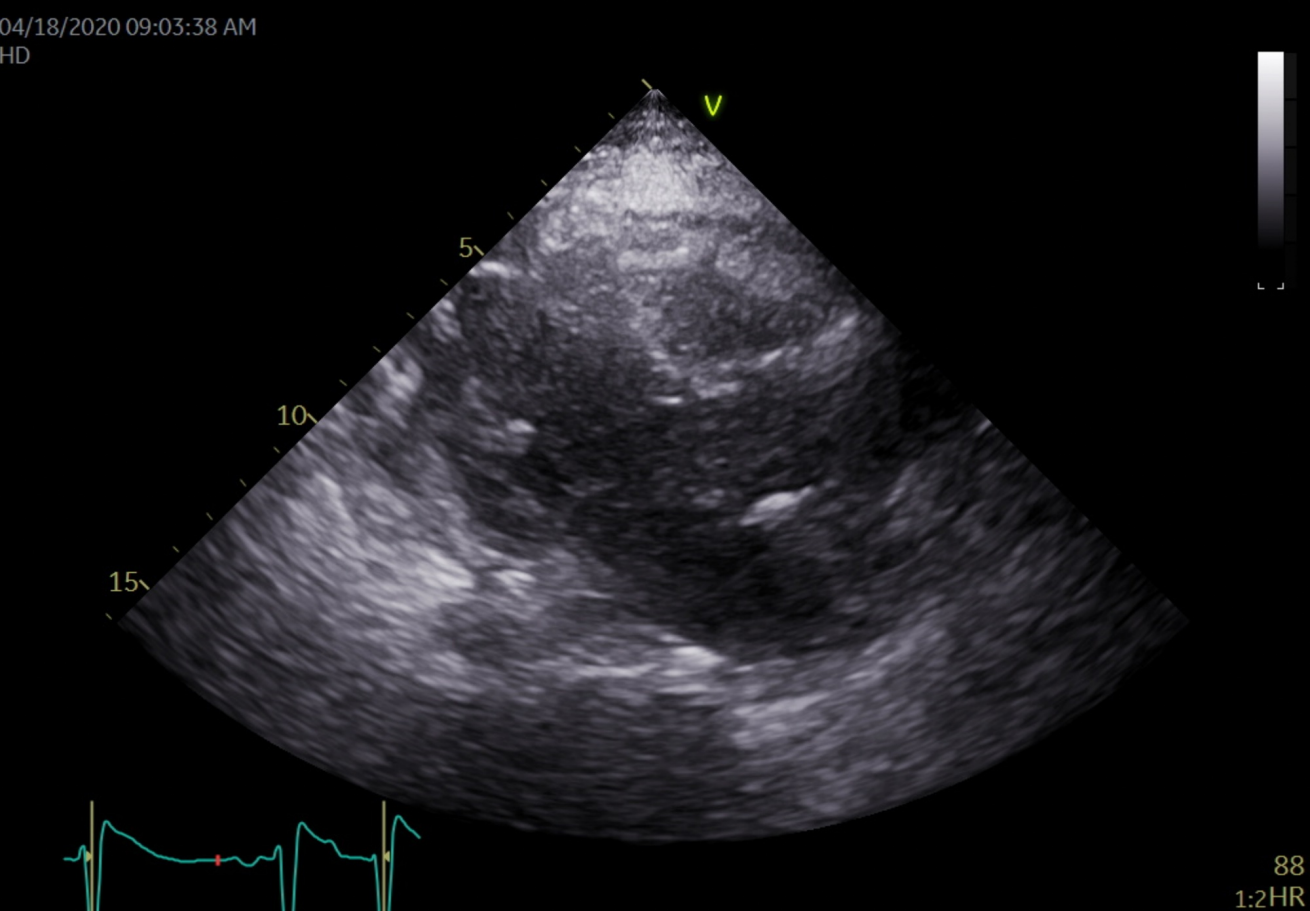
A parasternal long axis view showing the presence of the pericardial effusion that seems to be asymmetrically located closer to the right ventricle. This appears more consistent with malignancy or infection. There was no indication of tamponade physiology present here
